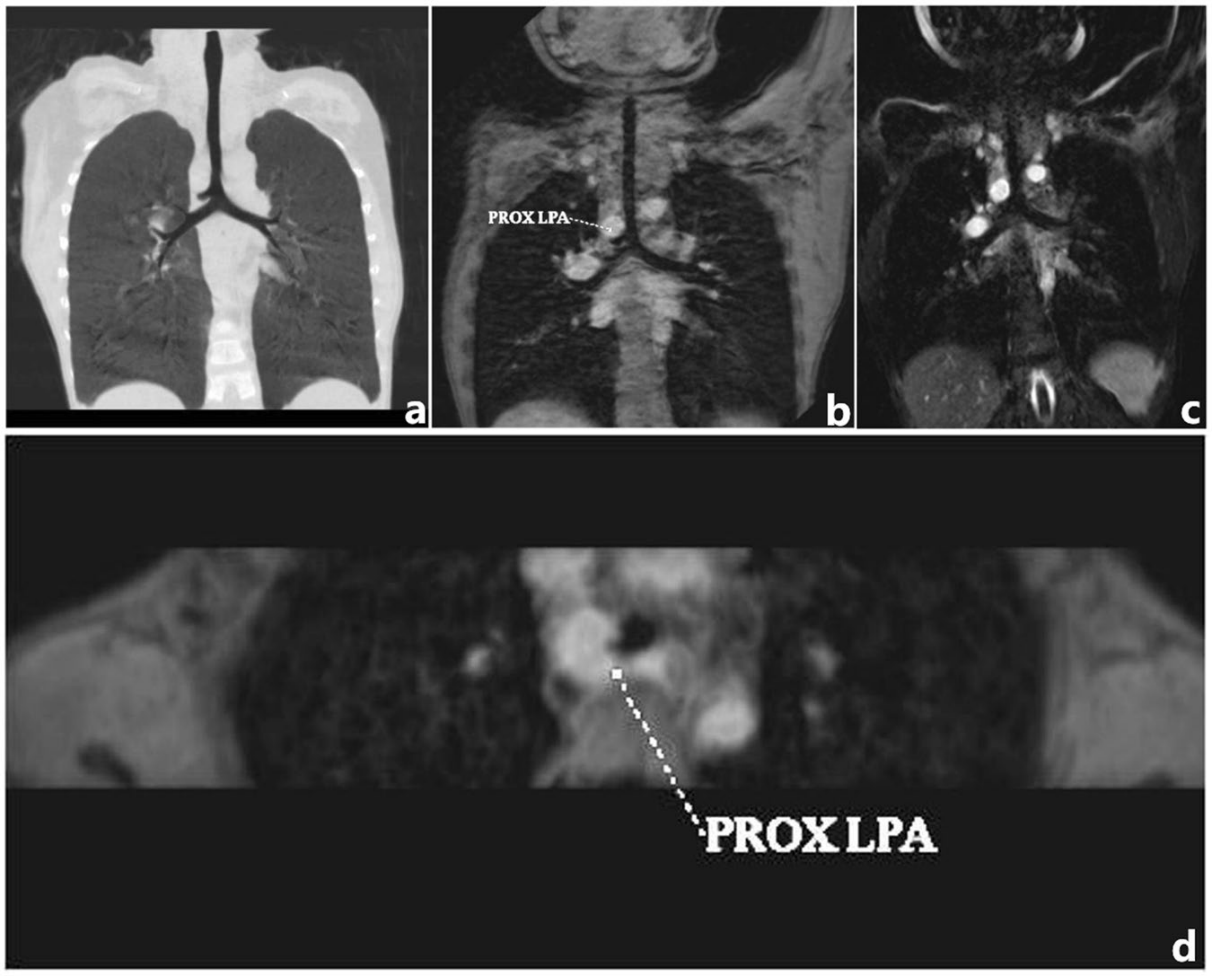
Six-year-old girl with left pulmonary artery sling. (a) CT coronal MinIP reconstruction demonstrated stenosis of the mild lower segment of the trachea and tracheal bronchus. (b) Three-dimensional turbo field echo (3D-TFE) coronal MinIP reconstruction demonstrated similar tracheobronchial tree findings. (c) Three-dimensional balanced TFE coronal MinIP reconstruction demonstrated similar findings, but the margin of the upper trachea was less clear than on 3D-TFE. (d) 3D-TFE axial MinIP reconstruction demonstrated that the left pulmonary artery arises from the right pulmonary artery and passes posteriorly between the trachea and esophagus.
